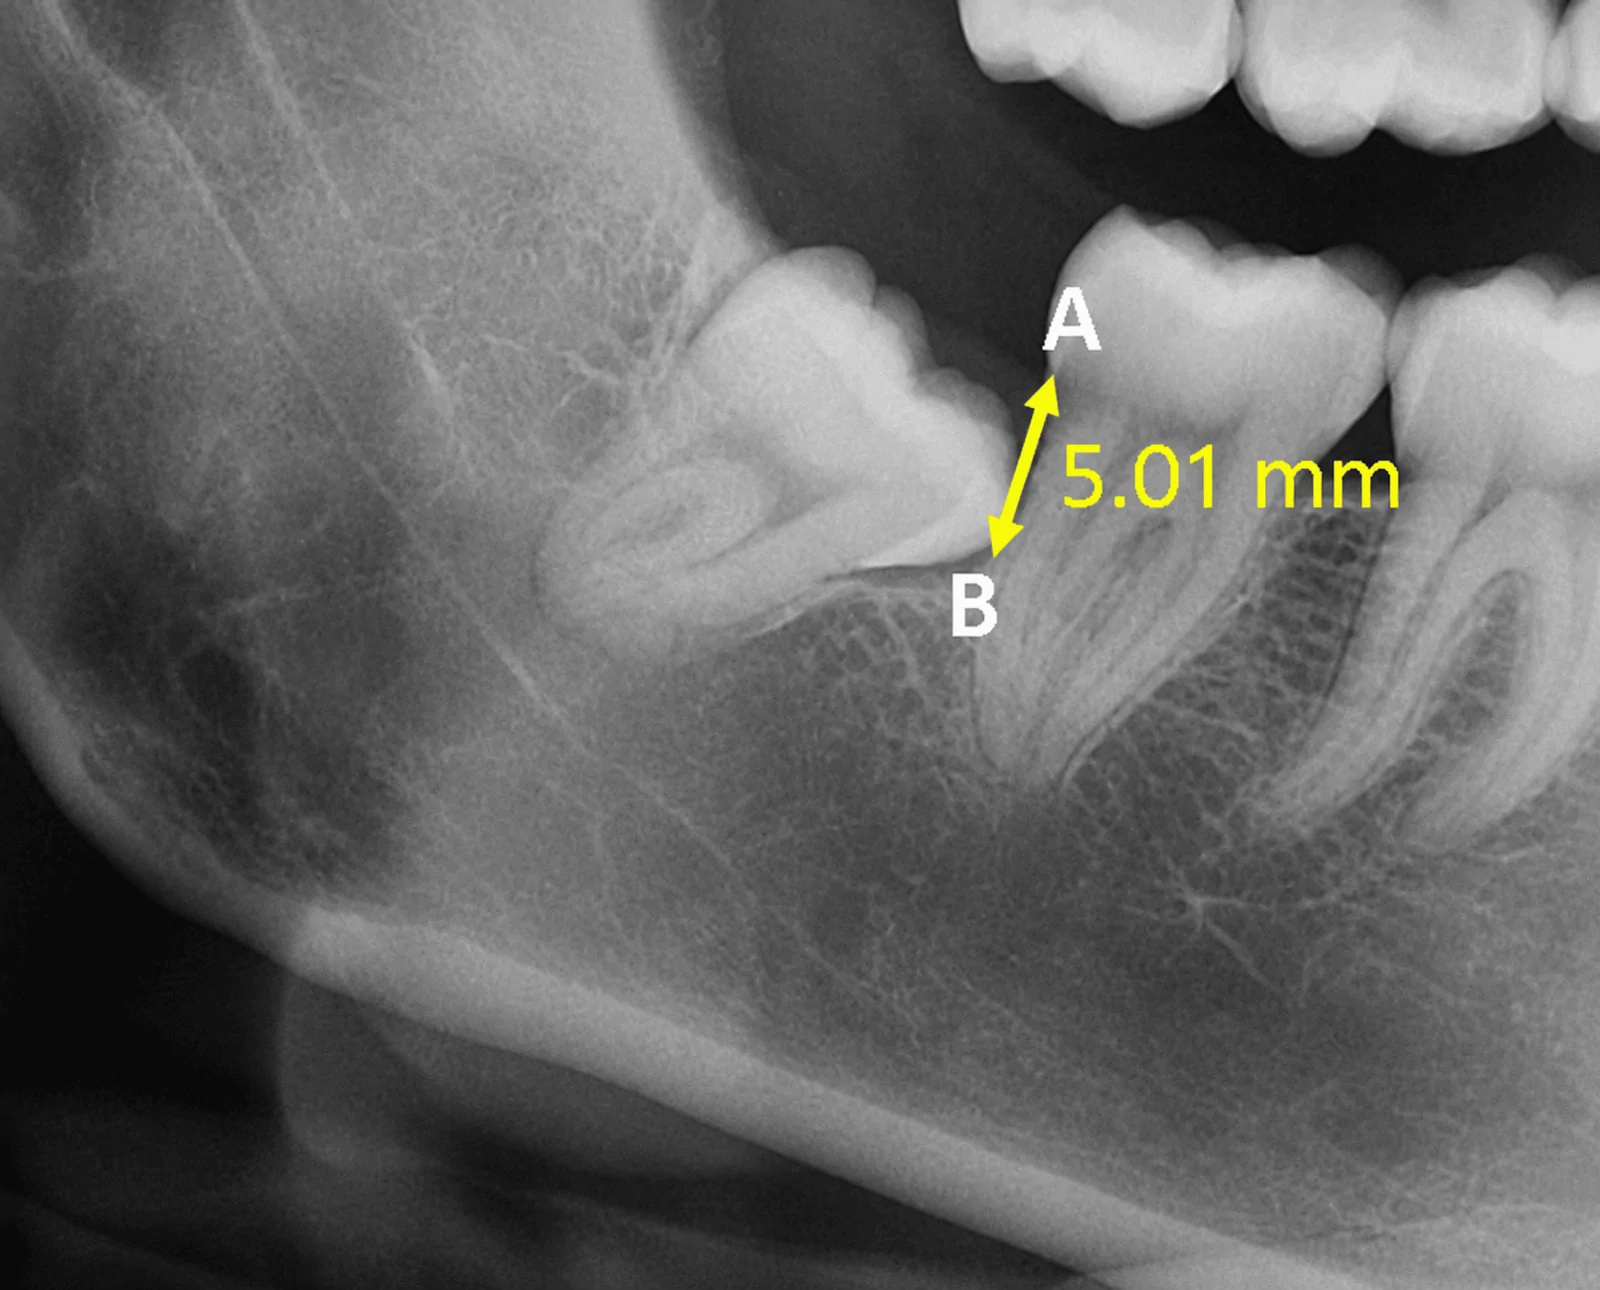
Measurement of the distal alveolar bone level on a panoramic radiograph. A: Distal cementoenamel junction (CEJ) of the mandibular second molar. B: Most coronal point of the distal alveolar crest. The measurement was performed as the linear distance between points A and B.
